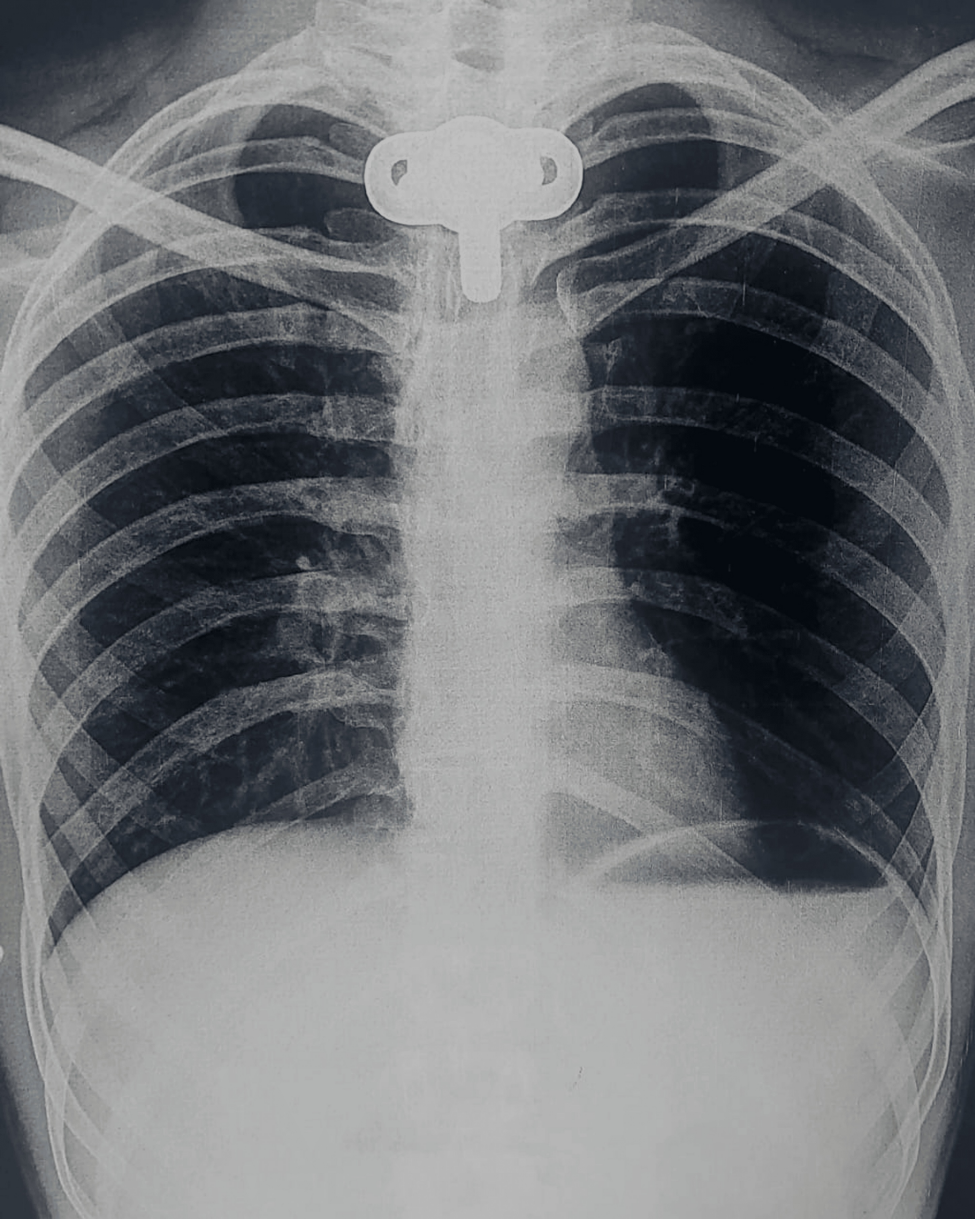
Chest X-ray on the day of admission Chest X-ray showed hyperinflated lung fields
